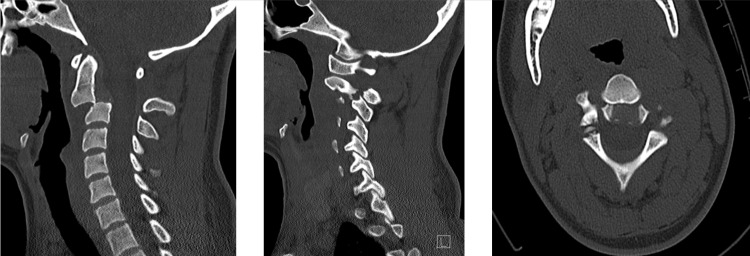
Preoperative CT scan Fracture of the posterior elements of C2 and pars interarticularis fracture of C2 extending through the vertebra on the left. The anterior endplate of the vertebral body was displaced by nearly its full width (at least 1 cm). Not pictured is the occlusion of the right vertebral artery on CT angiography.
